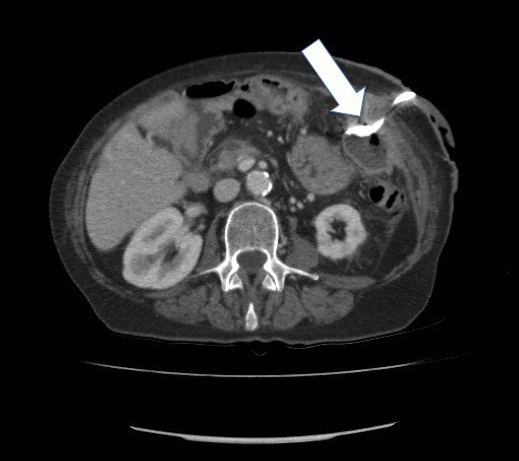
Axial portal venous phase contrast-enhanced CT: arrow shows decreased size of left upper quadrant collection with interval insertion of a pigtail drain.
